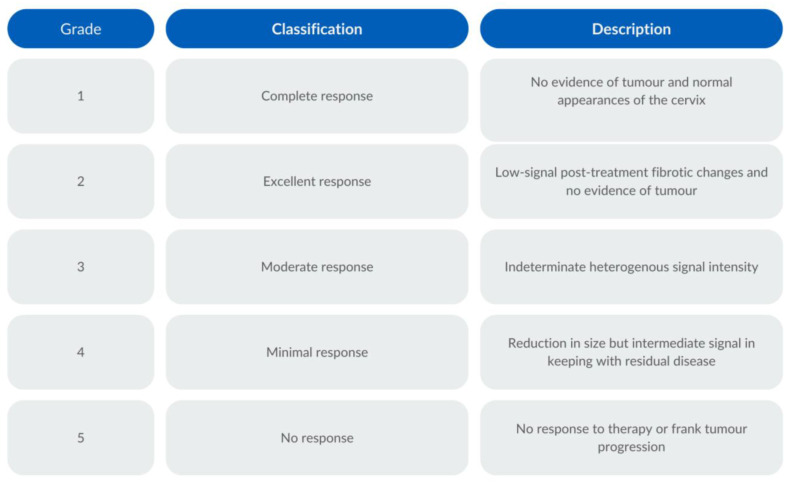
T2-weighted imaging response assessment scale.
